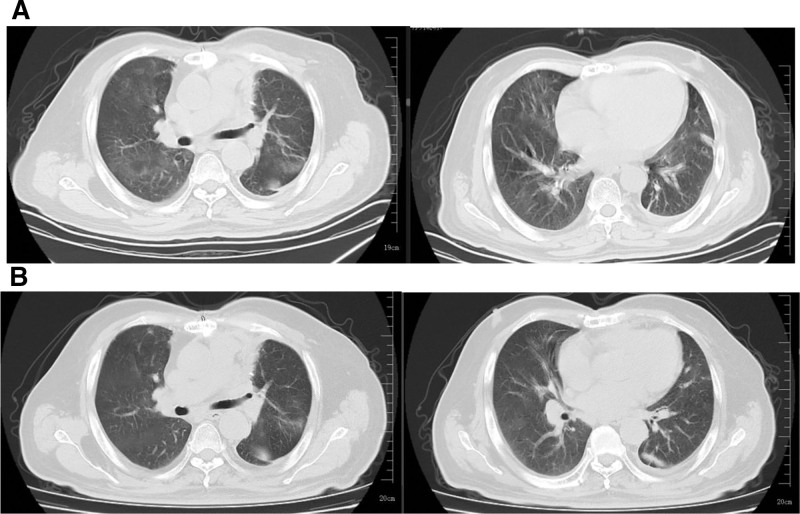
Chest CT images from the January 2023 episode: (A) on admission showing bilateral patchy ground-glass opacities and linear stranding and (B) 1 week post-discharge showing reduced size and fading of opacities. CT: computed tomography.
